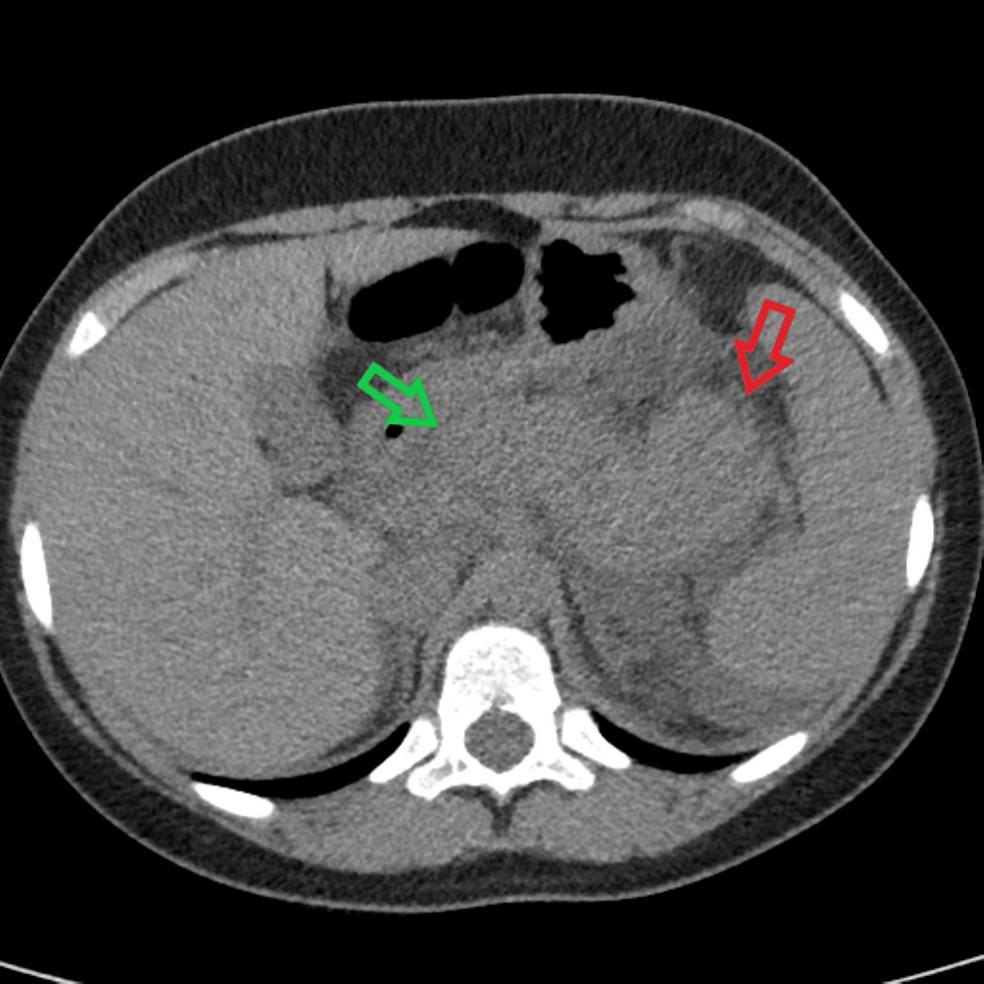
Non-contrast CT demonstrating the enlarged pancreas with extensive retroperitoneal and peripancreatic edema. An approximation of the head (green arrow) and the tail (red arrow) of the pancreas are shown. At the time of the study, it was noted that the lack of IV contrast limited the exam.
